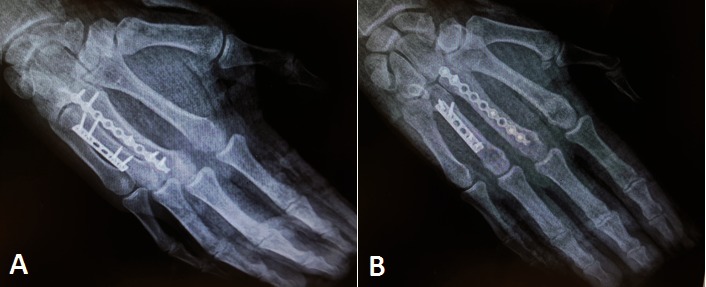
Oblique (A) and Plain (B) intraoperative radiographs
